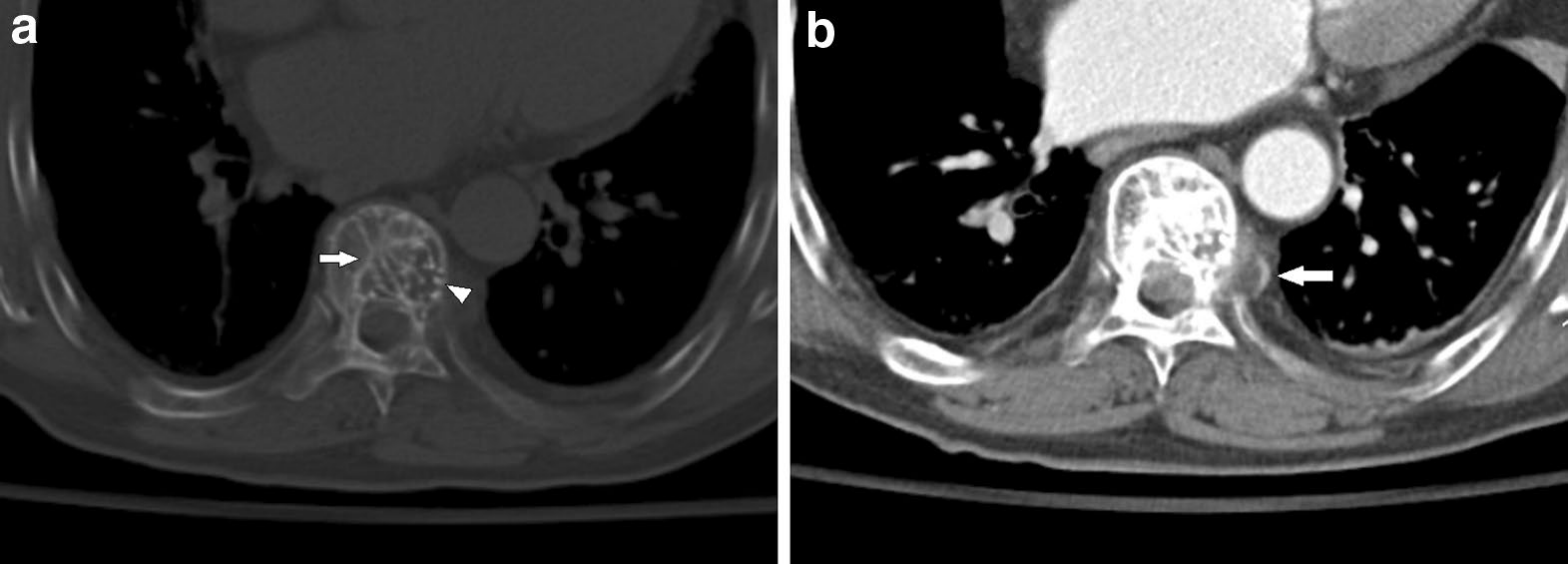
Case 2: a Unenhanced CT image at bone window settings shows that a lytic lesion with lattice-like coarse trabeculae, interrupted cortex (*arrowhead*), and partial peripheral sclerosis (*white arrow*). b Enhanced CT image shows paraspinal mass and posterior intercostal artery (*white arrow*) passing through the lesion without involvement
